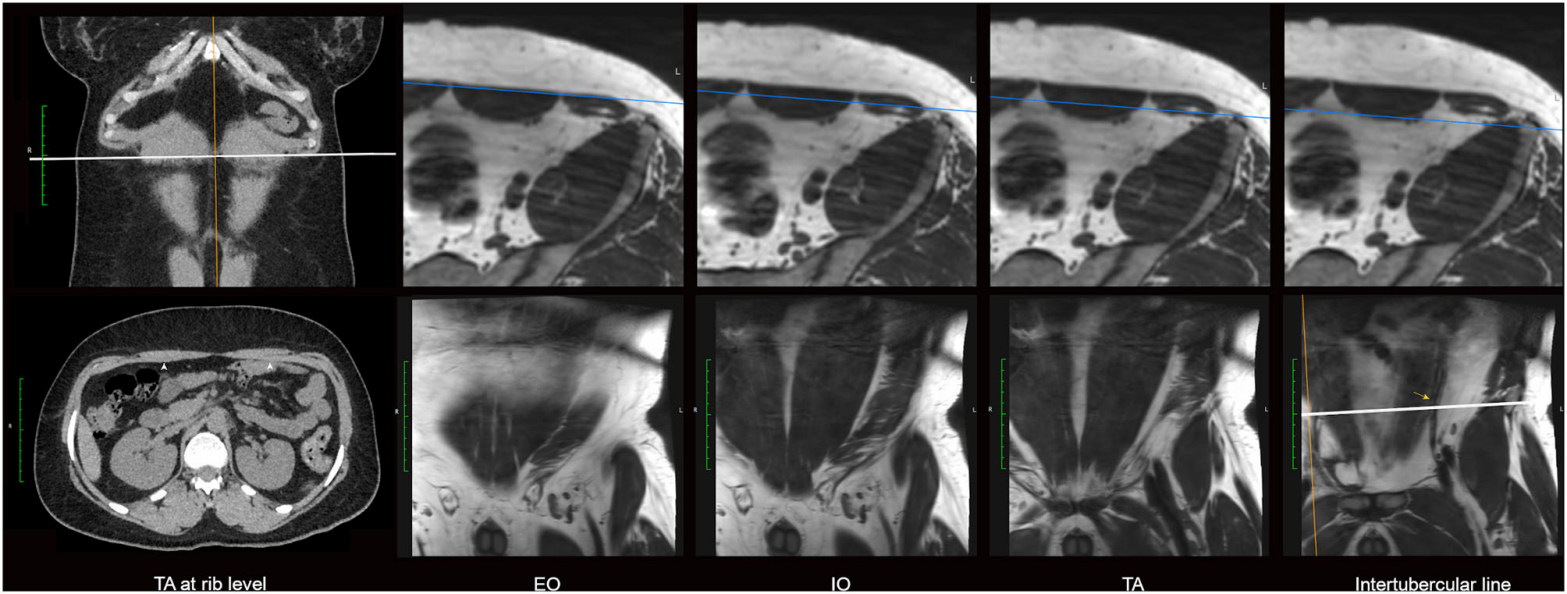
Anatomical arrangement of the upper & lower muscle segments of abdominal wall. CT images on the left show TA muscle (arrowheads) at rib level entering the rectus sheath posterior to each RA muscle. The remaining images are axial and sequential coronal oblique MRI sections obtained through the muscle layers of left lower quadrant abdominal wall in a male subject. Note the inferomedial long-axis fascicular orientation of all layers (EO, IO and TA) below the level of intertubercular line (indicated by white line on the far-right image of bottom row) toward the zone of pubic attachment and adductor cross-linkage. The arcuate line is not clearly resolved by imaging, but its approximate location can be suggested by the subtle angular change (yellow arrow) in the longitudinal course of the inferior epigastric vessels. The level of arcuate line varies between individuals but most commonly lies just above the intertubercular line.
